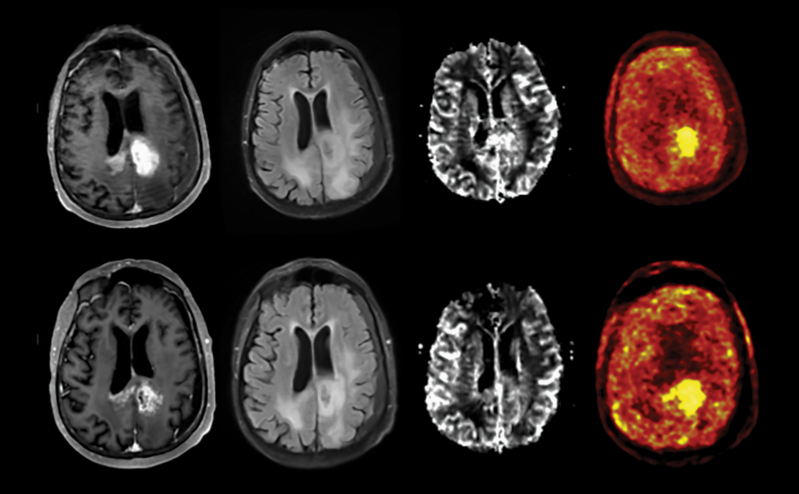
Example from one recurrent glioblastoma patient (FMS_01) demonstrating longitudinal change from baseline (top row) to week 3 (bottom row) with bevacizumab in contrast enhancement (A), T2/FLAIR (B), macroscopic CBV (C), and SUV map (D).
